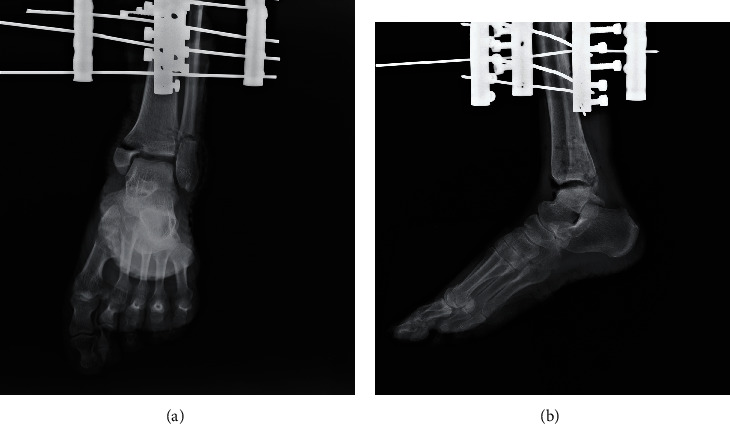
Radiographs were taken after modeling for the verification. A typical model's radiograph. (a) The anteroposterior view. (b) The lateral view.
